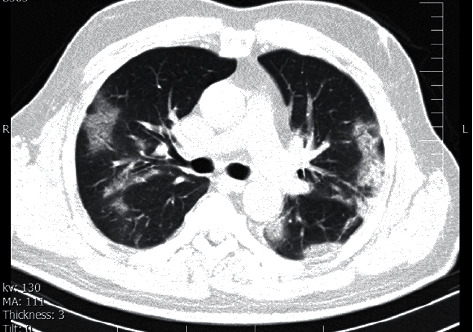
Axial chest CT image without contrast administration showed areas of ground-glass opacities and bilateral consolidations with the predominantly peripheral location.
